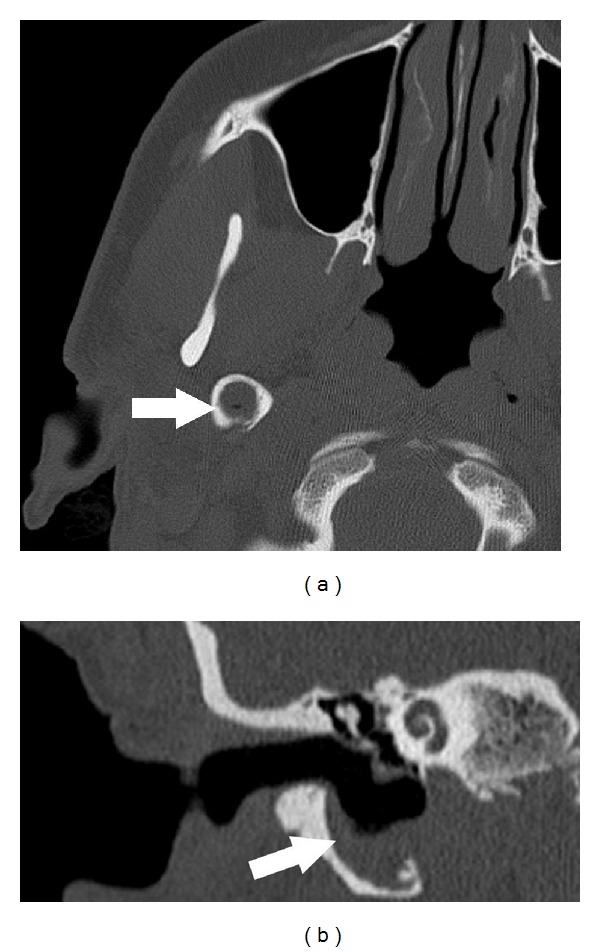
(a) Axial CT shows soft tissue attenuation material filling an expanded and pneumatised right styloid process (arrow), which on coronal reformat (b) communicates with an expanded hypotympanum.
